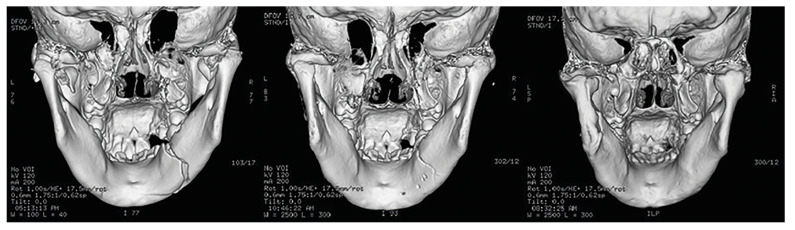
CT images of a case with severe dislocation of condylar fractures that underwent treatment of removing fracture fragments (left: before treatment; middle: 1 week after treatment; right: 6 months after treatment).
